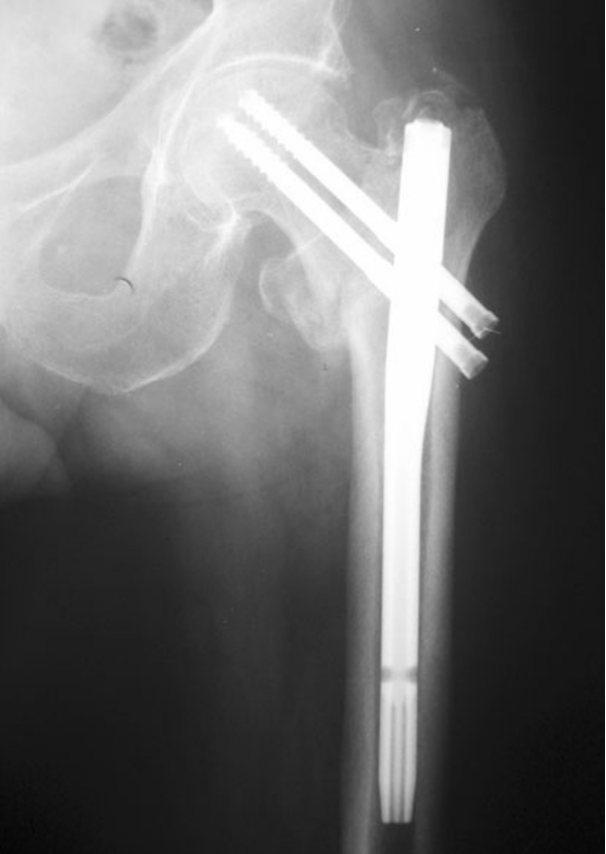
X-ray image showing a typical fracture with acceptable closed reduction, which eventually showed radiographically evident healing at the 4-month postoperative visit
